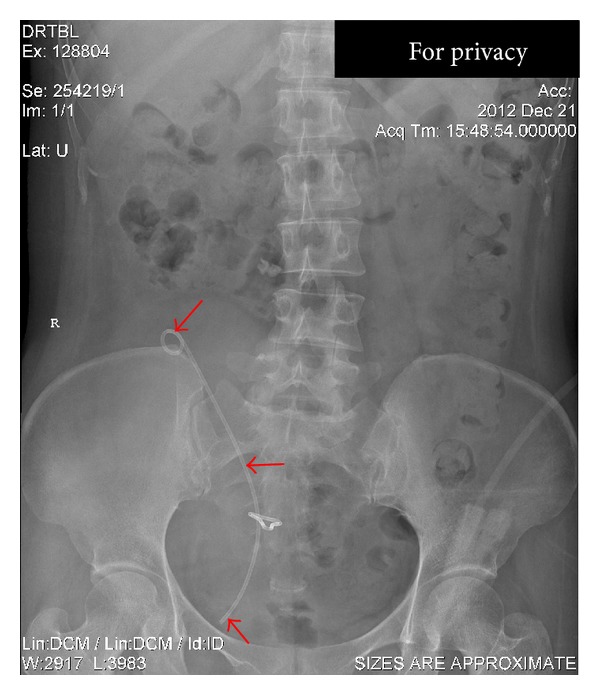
Preoperative kidney-ureter-bladder radiography image. The modified (by cutting a length of 9 cm from one end of the classical 25 cm full length) double J stent run from kidney to bladder was indicated with the red arrows.
